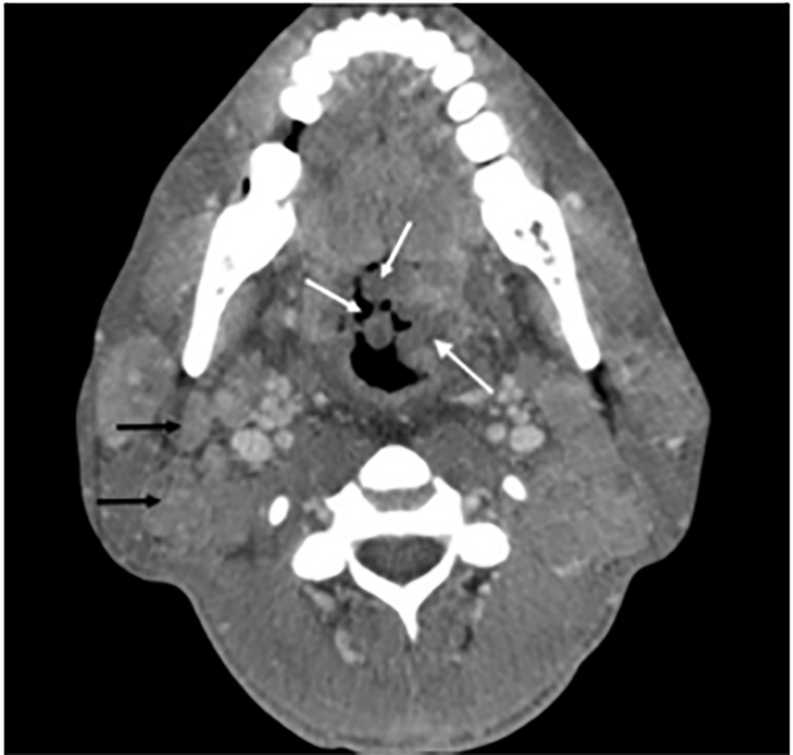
Axial computed tomography soft tissue neck showing diffuse nodular thickening of mucosal surfaces (white arrows) and extensive cervical lymphadenopathy (black arrows).
